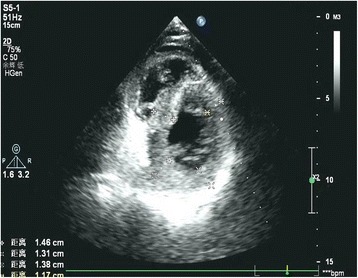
The echocardiographic study disclosed left ventricular thickening, “snowstorm” appearance of the myocardium and a small amount of excessive pericardial fluid
